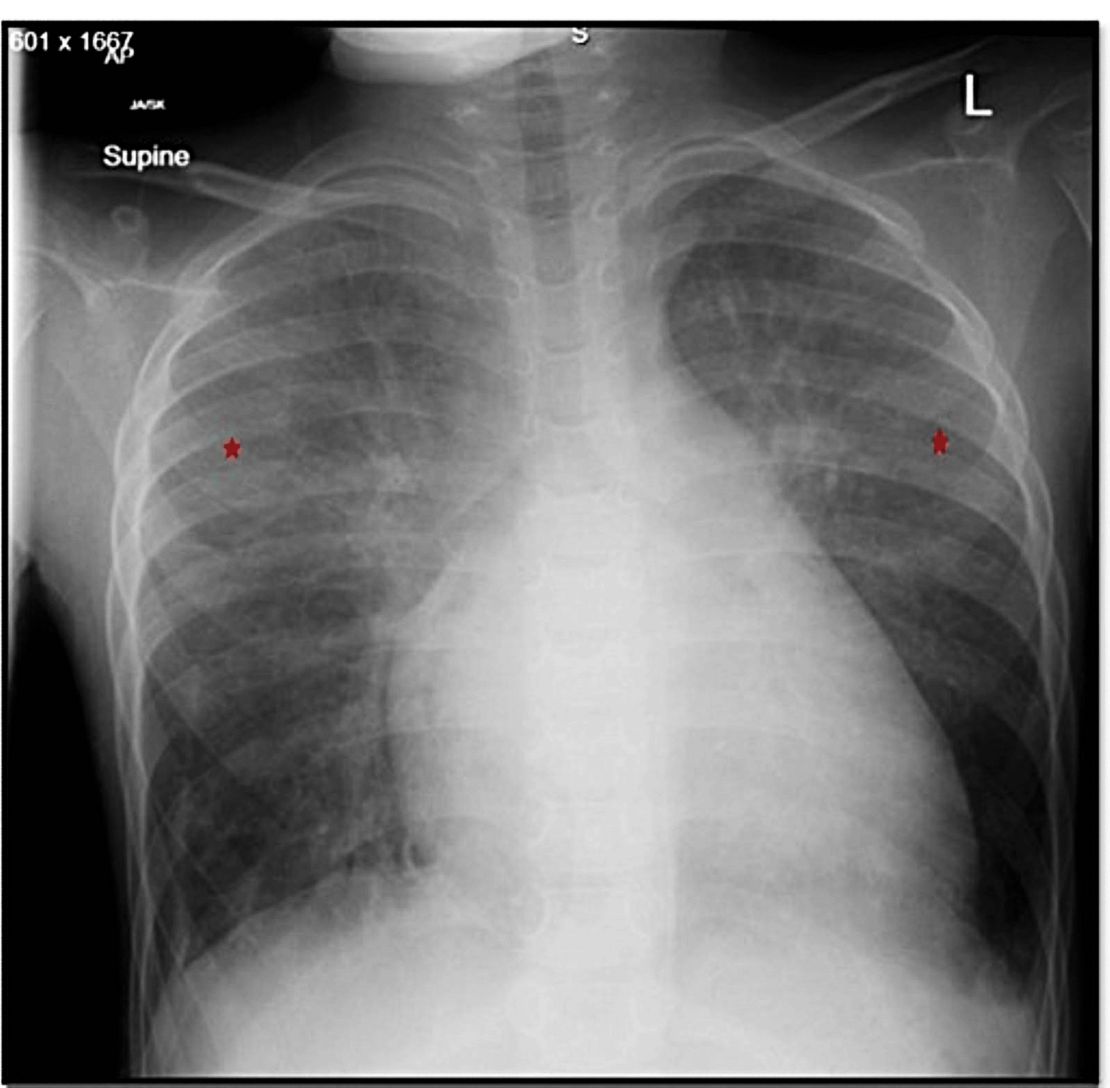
Anteroposterior chest X-ray showing diffuse hazy opacification of the lung parenchyma (red asterix) relatively sparing the peripheral lung bases with medial lung atelectasis.
